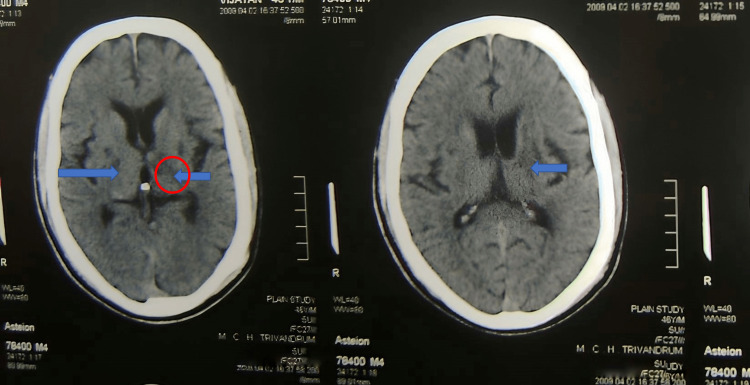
CT brain showing bilateral infarcts in the posterior limb of internal capsule (shown in blue arrow and red circle).
